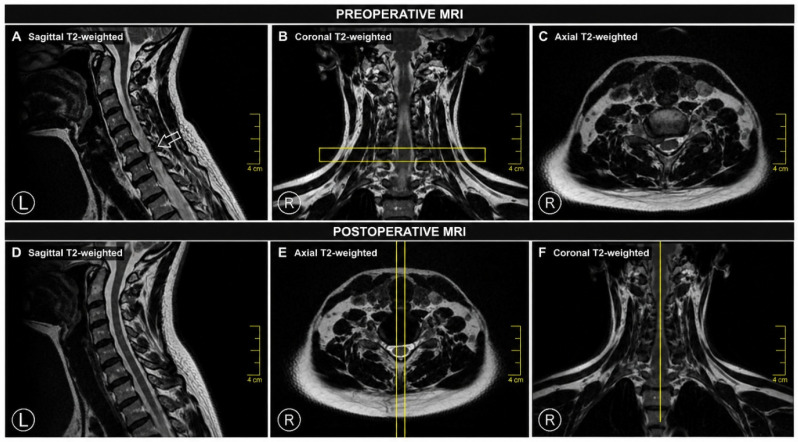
Representative preoperative and postoperative MRI findings after combined posterior endoscopic cervical diskectomy and foraminotomy. (A–C) Preoperative sagittal, coronal, and axial T2-weighted MRI images demonstrating unilateral cervical foraminal stenosis with concomitant lateral soft disc herniation and compression of the exiting cervical nerve root. The white arrow in panel A indicates the lateral soft disc herniation at the C6/C7 level, and the yellow box in panel B indicates the corresponding C6/C7 disc level in the coronal plane. (D–F) Postoperative sagittal, axial, and coronal T2-weighted MRI images obtained after combined posterior endoscopic cervical discectomy and foraminotomy, demonstrating increased foraminal caliber and visible decompression of the exiting nerve root, with no evident residual nerve root compression on postoperative MRI. The yellow midline reference markers in panels E and F indicate the median anatomical plane and serve as orientation landmarks for postoperative imaging assessment. MRI—magnetic resonance imaging.
